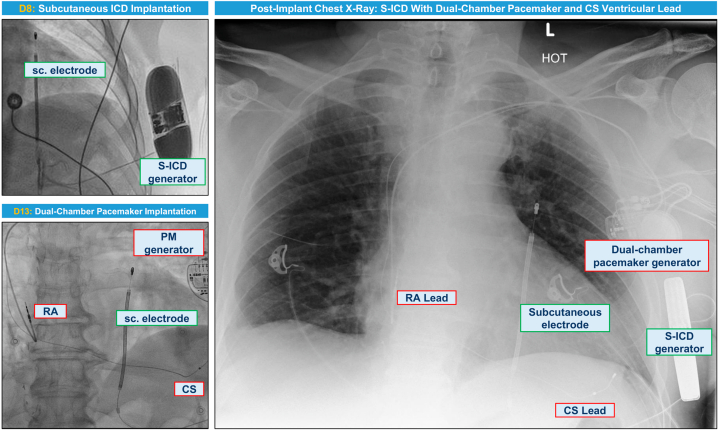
Device Therapy Combining S-ICD and Transvenous Atrial Plus Coronary Sinus Ventricular Pacing Procedural fluoroscopy and postimplant chest radiograph documenting the combined device configuration: S-ICD implanted on hospital day 8, followed by dual-chamber pacemaker implantation with a coronary sinus ventricular lead on hospital day 13 after onset of sinus arrest. CS = coronary sinus; ICD = implantable cardioverter-defibrillator; PM = pacemaker; RA = right atrium; sc. = subcutaneous; S-ICD = subcutaneous implantable cardioverter-defibrillator.
